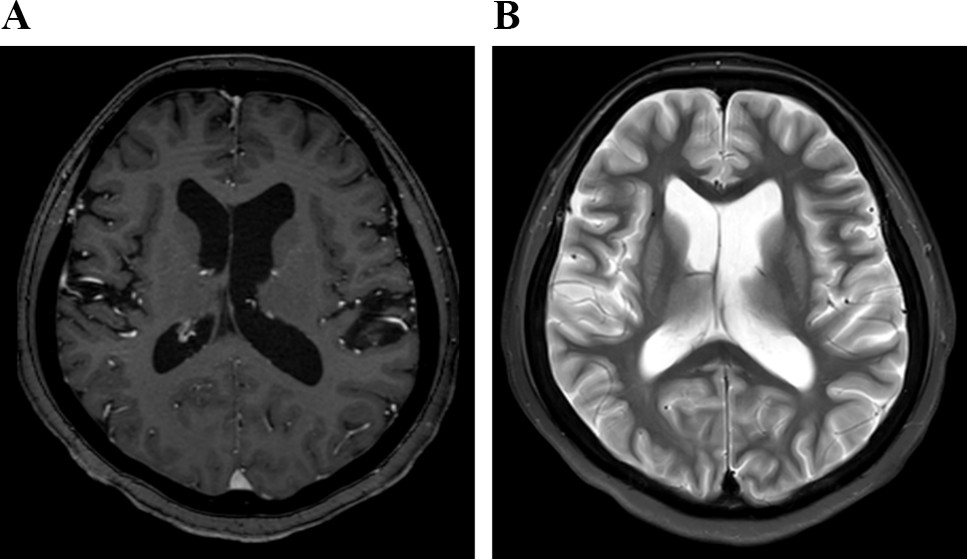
Case 11 (giant cell arteritis). (a), Contrast-enhanced T1-weighted (CE-T1W) revealed no abnormal signal area. (b), STIR revealed no abnormal signal area
